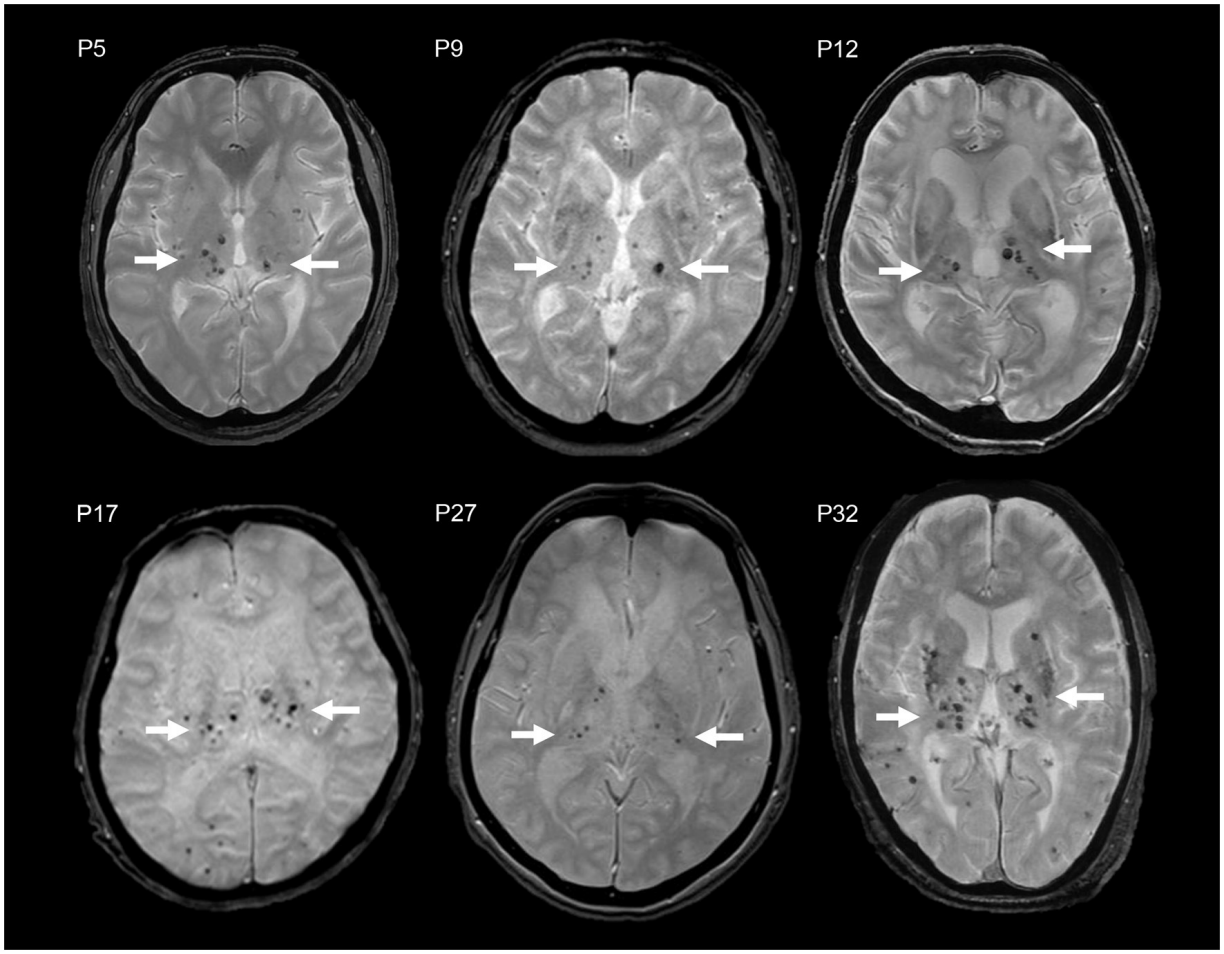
MCT signs in brain MRI T2*WI of six CADASIL patients with R75P mutation (P5, P9, P12, P17, P27, and P32). Multiple CMBs are accumulated in the thalamus (arrow).
